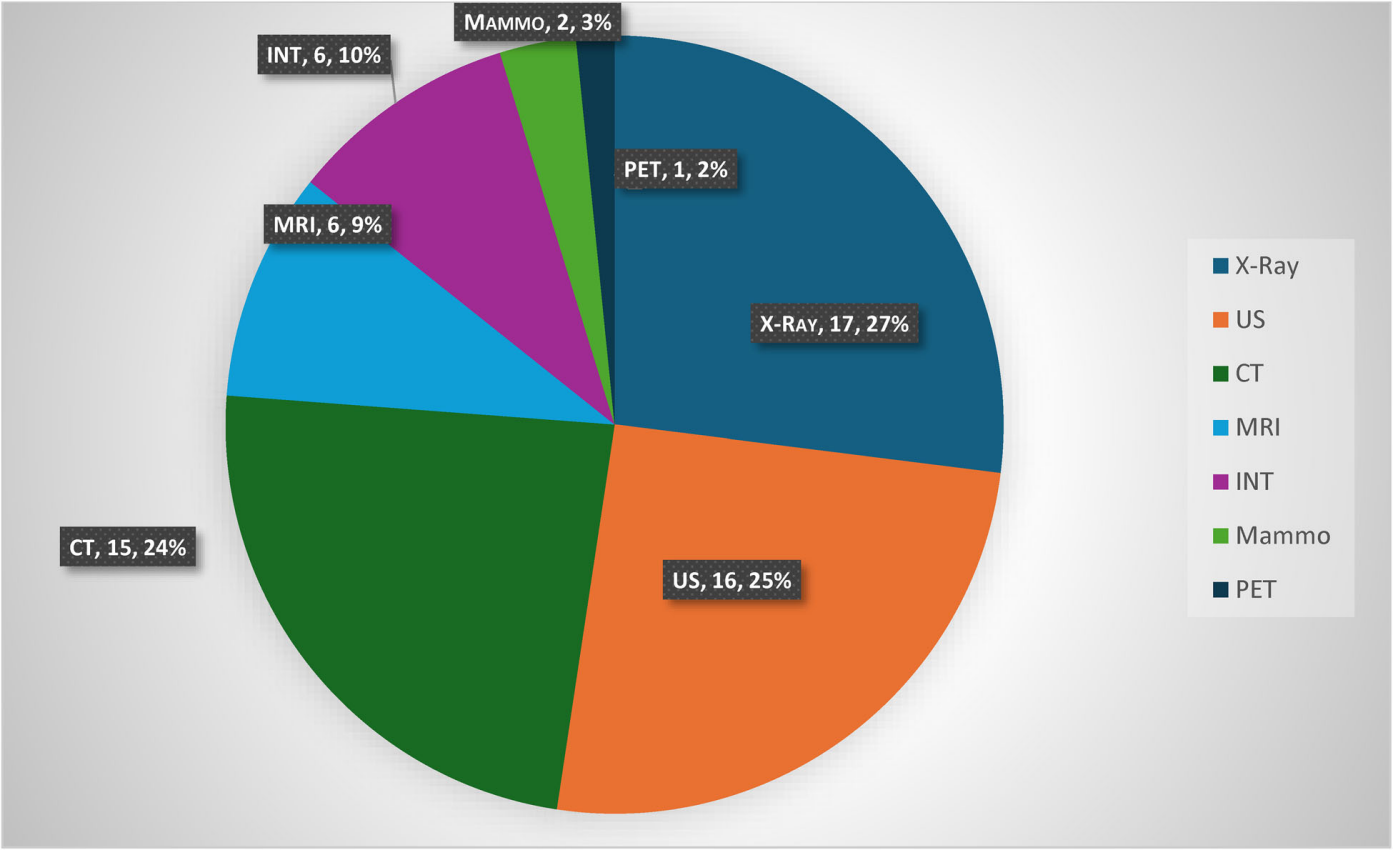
Non‐indicated imaging request types as reported by participants. INT, intervention; CT, computer tomography; MRI, magnetic resonance imaging; PET, positron emission tomography; Mammo, mammography; US, ultrasound.
